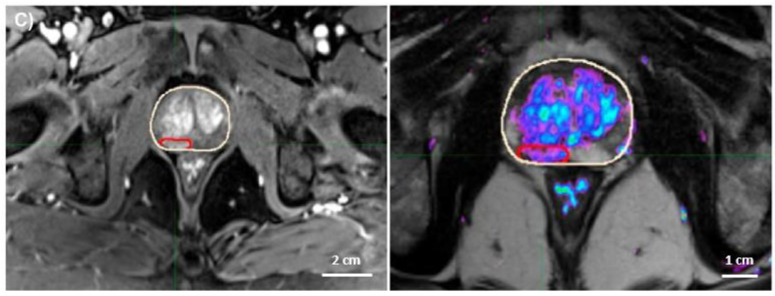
(A,B) The putative tumor cells overexpressing all three antigens of interest (PSMA, CK, and GPC-1) were considered CTCs. At the same time, a high number of the putative tumor cells significantly positive for one or two of the three antigens was also observed in the patients’ samples, representing potential antigen heterogeneity of the PCa tumor. (C) The MRI scan of one of the PCa patients’ prostates (white circle) showing the tumor (red circle) as the potential source of CTCs in the seminal fluid.
